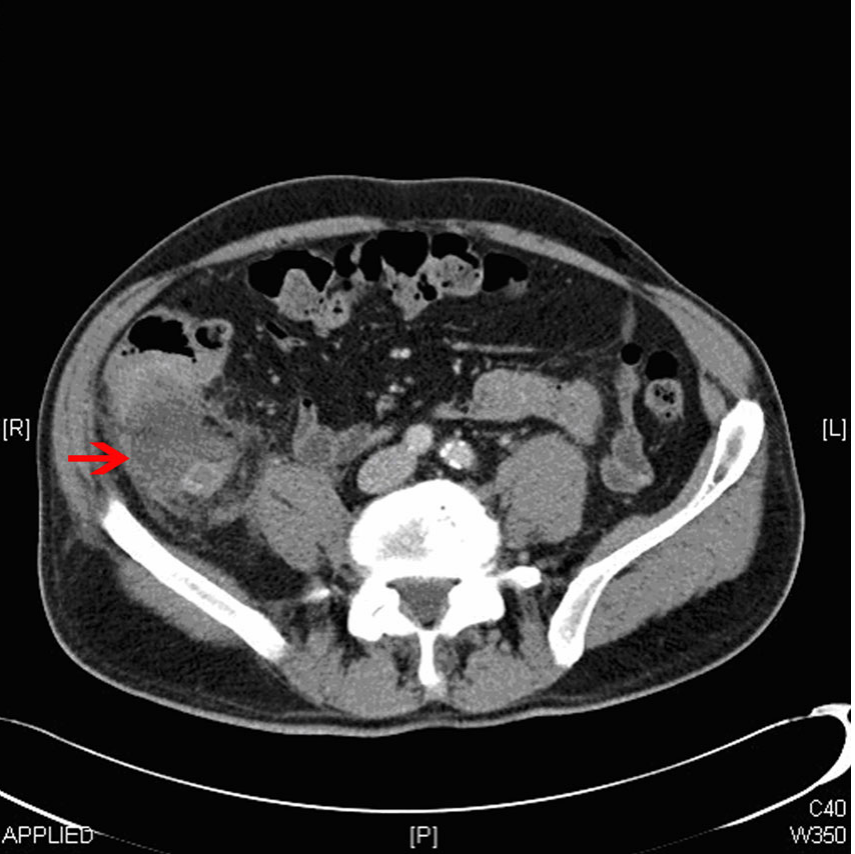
Computed tomography scan showing appendix mucocele.
